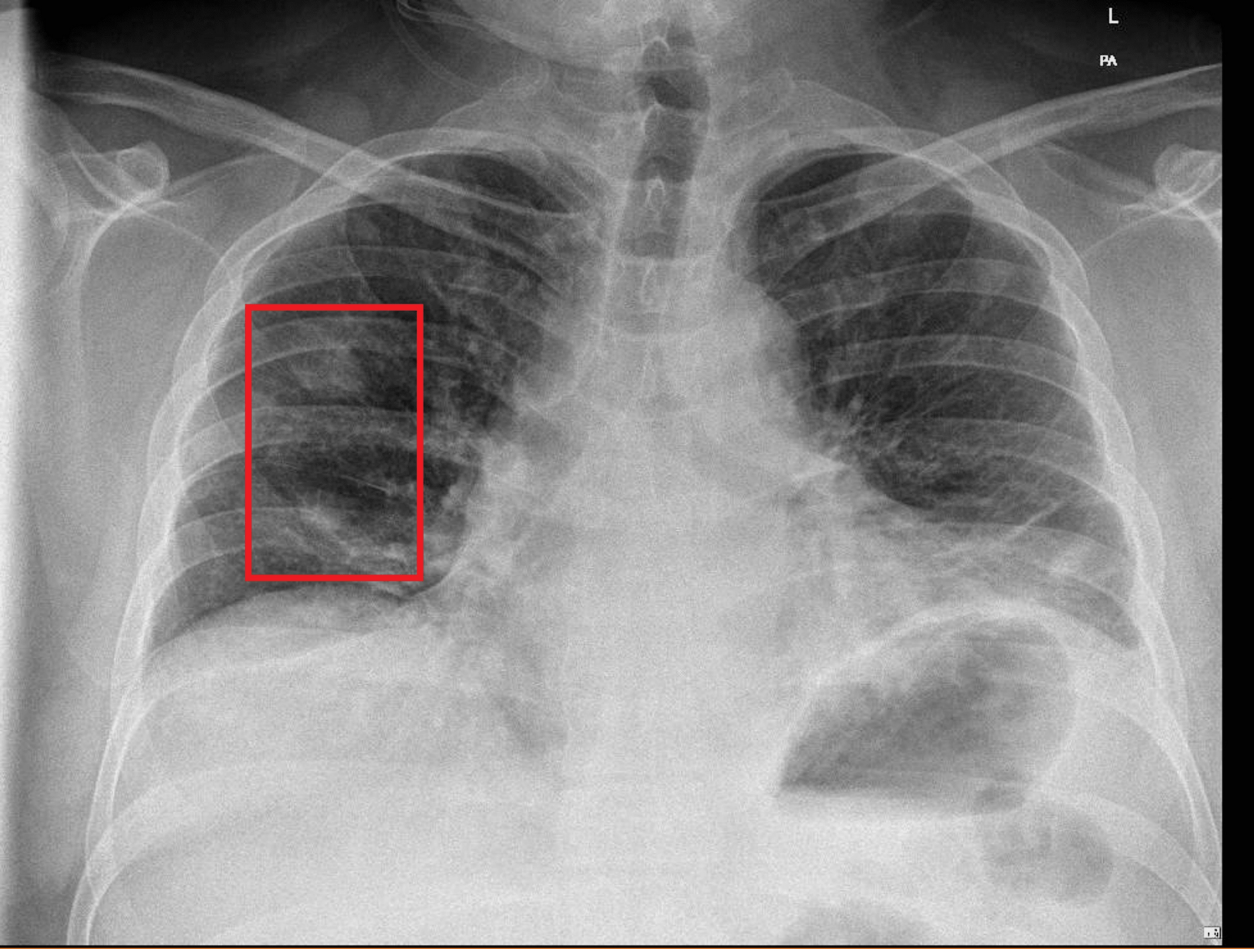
Chest X-ray on admission showing right middle zone opacity
